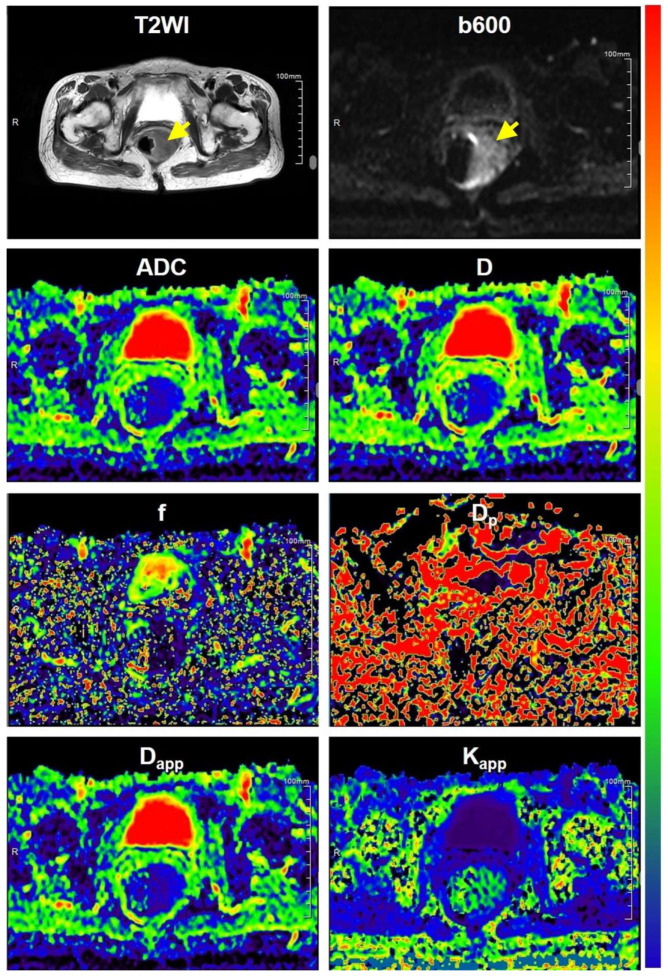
Representative MR images of a patient with high grade rectal cancer. Notes: All parametric maps employed a consistent color bar, spanning from blue to red, to signify the minimum and maximum values, respectively. The parametric ranges for ADC, D D_p_, f, D_app_, K_app_ are as follows: 0.0 to 5.0 × 10^−3^ mm^2^/s, 0.0 to 5.0 × 10^−3^ mm^2^/s, 0.0 to 80.0 × 10^−3^ mm^2^/s, 0.0 to 0.8, 0.0 to 5.0 × 10^−3^ mm^2^/s, 0.0 to 2.5, respectively. The yellow arrows indicate the tumors.
